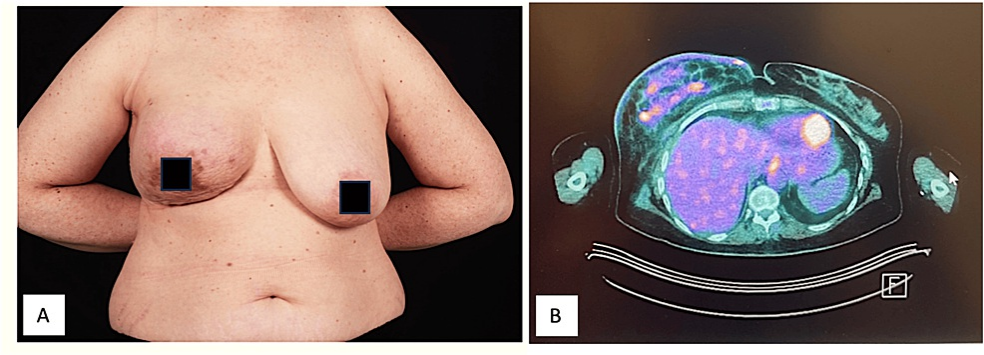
Inflammatory right breast cancer. A: Pre-operative right inflammatory breast cancer after seven cycles of adjuvant chemotherapy. B: PET-CT post-neoadjuvant chemotherapy.
